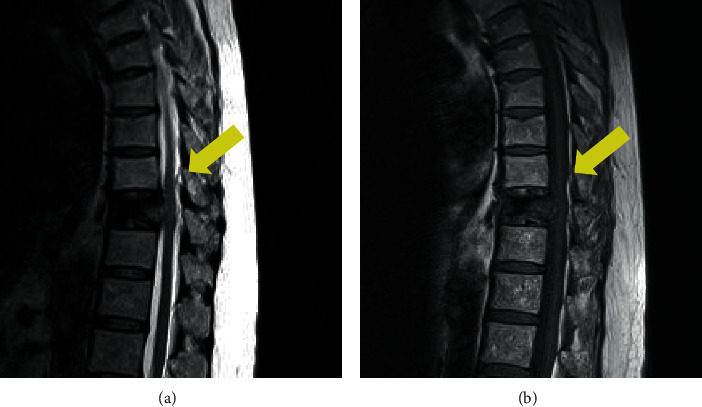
Sagittal views of thoracic magnetic resonance images on T2-weighted images (a) and T1-weighted images (b). T10 vertebral body demonstrated low intensity on the T2- and T1-weighted images and small epidural mass (yellow arrow) at the T9 dorsal side of the central canal was hyperintense on the T2-weighted images and was iso- to hyperintense on the T1-weighted images. These findings indicate T10 vertebral fracture and epidural hemorrhage.
